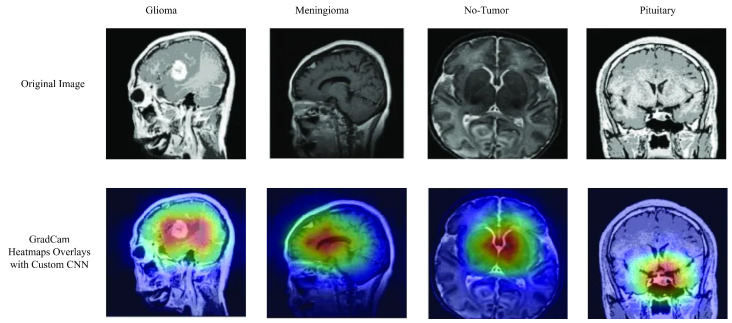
Grad-CAM visualizations highlighting discriminative regions in MRI scans, demonstrating that the model focuses on anatomically relevant tumor regions for decision-making.
